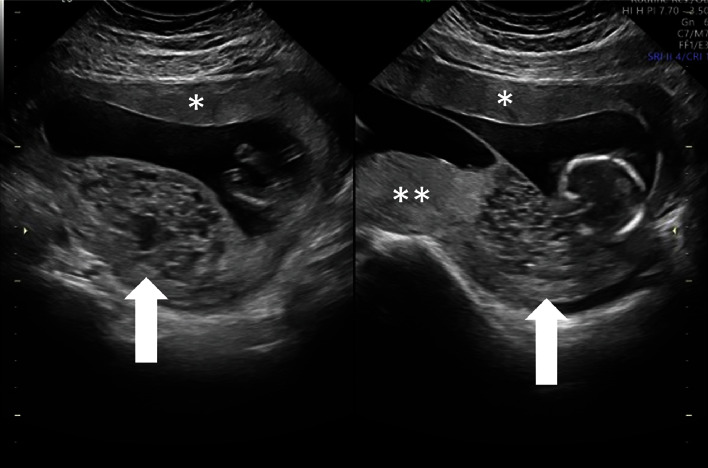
Ultrasound at 21 weeks showing three separate placentas and a dividing membrane ∗Twin A placenta. ∗∗Twin B placenta. Up arrow: molar placenta.
